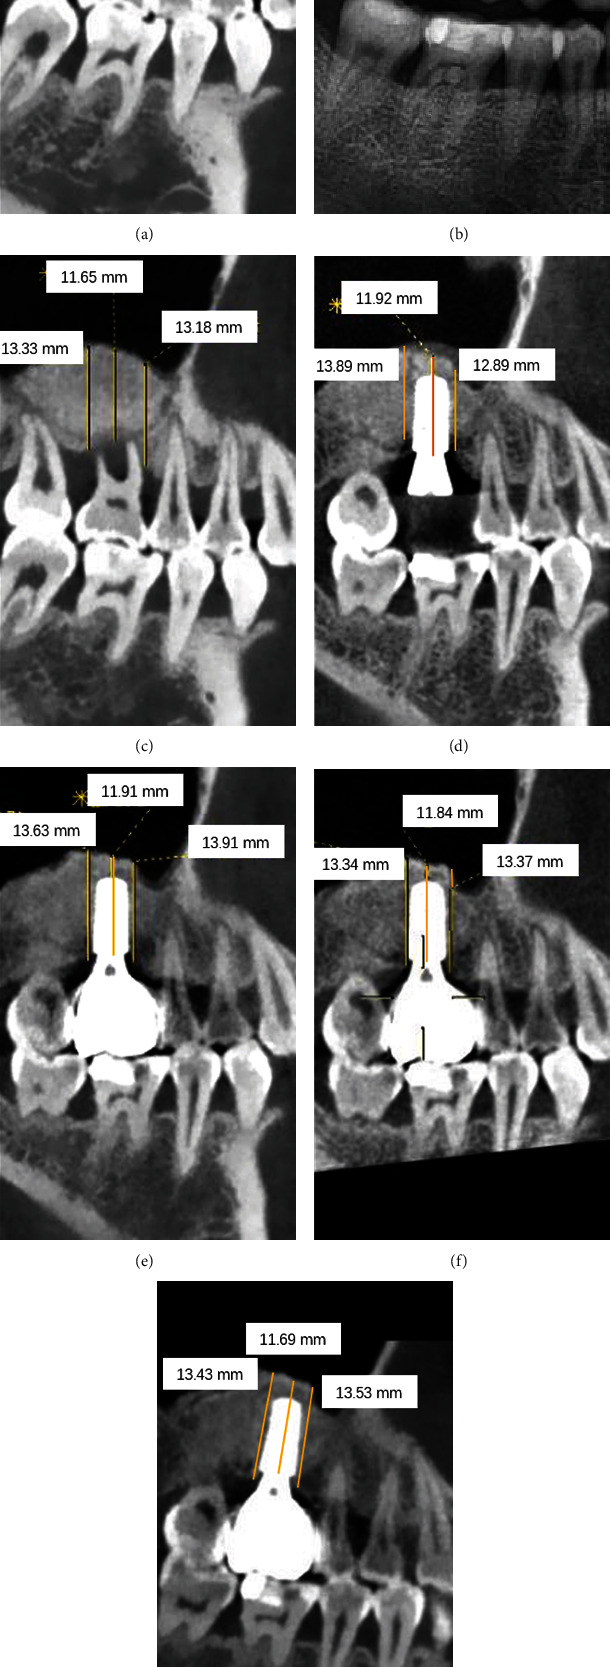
CBCT and pantomograph follow-up for tooth #16. (a) Before MSFE. (b) Immediately after MSFE (pantomograph). (c) 3 months after MSFE. (d) 10 months after MSFE. (e) 15 months after MSFE. (f) 26 months after MSFE. (g) 35 months after MSFE.
